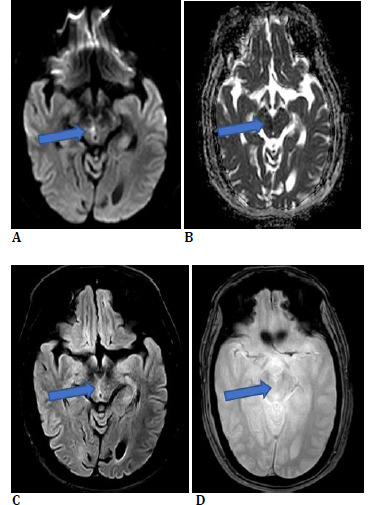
MRI brain at the level of upper mid brain (superior colliculus) showing (A) tiny focus of mild diffusion restriction on diffusion weighted imaging (B) with corresponding low ADC value (C), which shows FLAIR mismatch suggestive of hyperacute infarct at right paramedian location; (D) No blooming on gradient echo images
